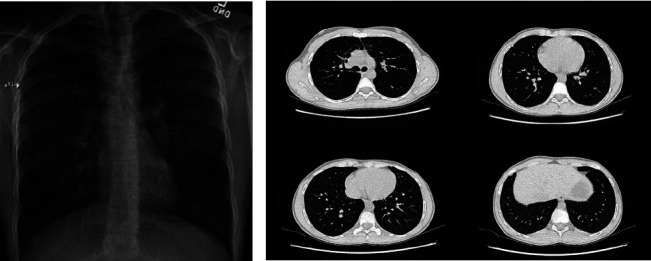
(a) Posterior-anterior chest X-ray reveling diffuse, fine reticulonodular haziness. (b) Lung-window computed tomography (CT) scan revealing small cystic spaces (0.2–0.4 millimeters) and ground-glass micronodularity.
